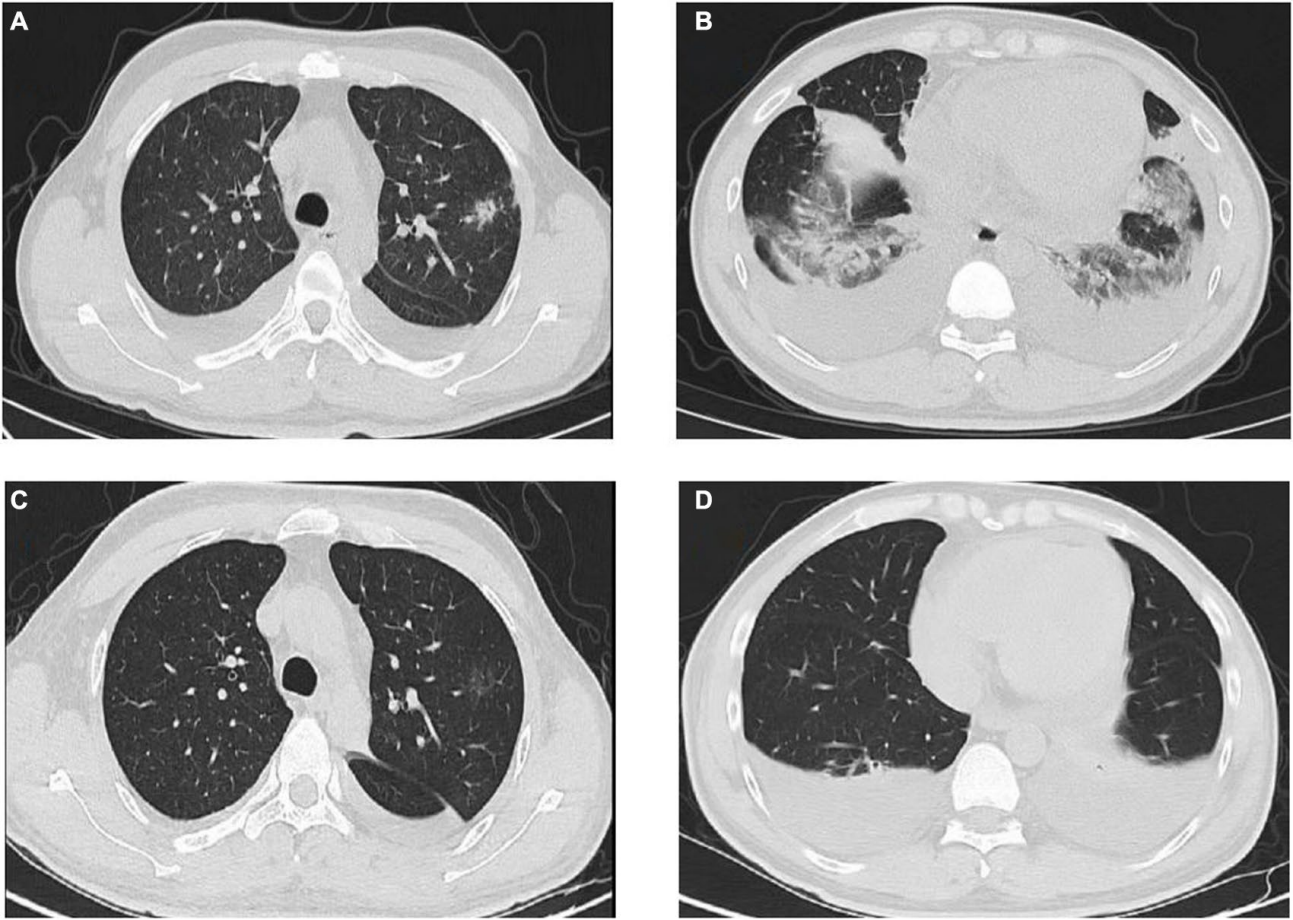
Comparison of before and after lung CT results. (A,B) February 22nd lung CT: Multiple solid nodules with exudation in the posterior segment of the left upper lobe of the lung, and exudation in the lower lobes of both lungs. (C,D) March 30th lung CT: The inflammatory nodules in the upper lobe of the lung were significantly absorbed and became lighter, and some were completely absorbed; bilateral pleural effusion.
